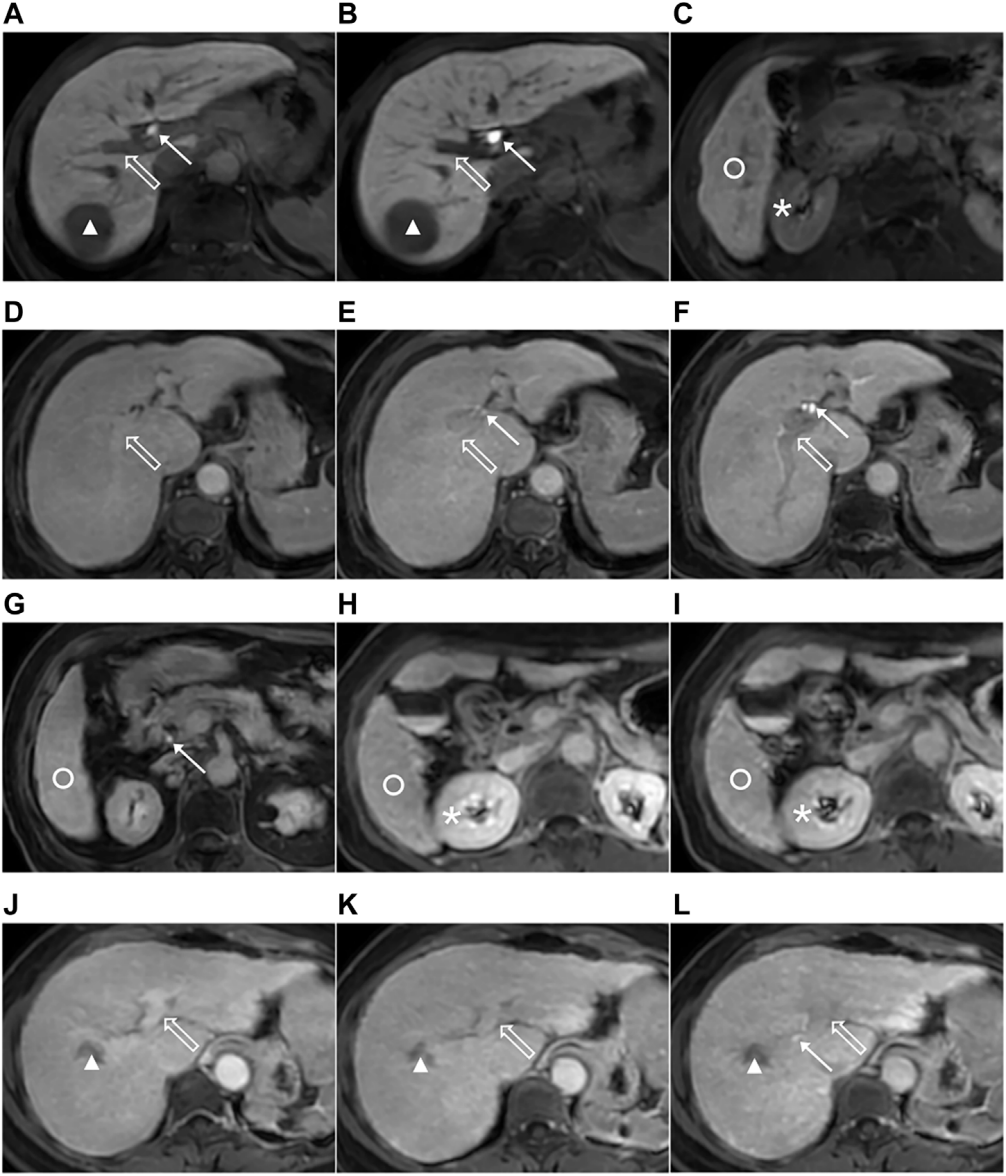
Images from 3.0-T MRI that were obtained 5, 10, and 15 min after gadoxetic acid was administrated within axial planes in the three patients that suffer from the chronic liver disease or the liver cirrhosis. The 51-year-old man that suffer from the chronic hepatitis B, hepatocellular carcinoma (HCC) (triangle), ICG-R_15_ of 3.6%, ALBI grade 1, CP A cirrhosis and MELD score of 8 with the functional liver imaging score (FLIS) of 6 at 5 (A,C), 10 (B) and 15 min (not shown). Liver parenchymal enhancement quality score (EnQS) is 2 at each time point due to that the signal intensity of the liver (circle) is higher compared to that of the right kidney (*). Excretion quality score (ExQS) is 2 at each time point due to that the contrast media is found to be within the common bile duct (solid arrow). Portal vein sign quality score (PVsQS) is 2 at each time point due to that the portal vein (hollow arrow) is seen to be hypointensed to the liver parenchyma (circle). A 78-year-old woman with cryptogenic liver disease, HCC (not shown), ICG-R_15_ of 6.8%, ALBI grade 2, CP A cirrhosis and MELD score of 15 with functional liver imaging score (FLIS) of 2, 3 and 5 at 5 (D), 10 (E) and 15 min (F,G), respectively. EnQS is 1 at each time point due to that there is equal signal intensity of the liver (circle) and the right kidney (*). ExQS is 0, 1, and 2 at 5, 10, and 15 min, respectively, due to that contrast media is not presented, excreted into the intrahepatic bile ducts and common bile duct respectively (solid arrow). PVsQS is 1 at 5 and 10 min and 2 at 15 min due to that the portal vein (hollow arrow) is found to be both isointense and hypointense to the liver parenchyma (circle), respectively. A 59-year-old woman that suffers from the hepatitis C virus cirrhosis, HCC (triangle), ICG-R_15_ of 21.0%, ALBI grade 2, CP A cirrhosis and MELD score of 7 with the functional liver imaging score (FLIS) being 0, 1 and 3 at 5 (J), 10 (H,K) and 15 min (I, L), respectively. EnQS is 0 at 5 and 10 min and 1 at 15 min due to that the signal intensity of the liver (circle) is both lower than and equals to that of the right kidney (*), respectively. ExQS is 0 at 5 and 10 min and 1 at 15 min because contrast media is not shown and excreted into the intrahepatic bile ducts (solid arrow). PVsQS is 0 at 5 min and 1 at 10 and 15 min due to that the portal vein (hollow arrow) is both hyperintens and isointense to the liver parenchyma (circle), respectively.
